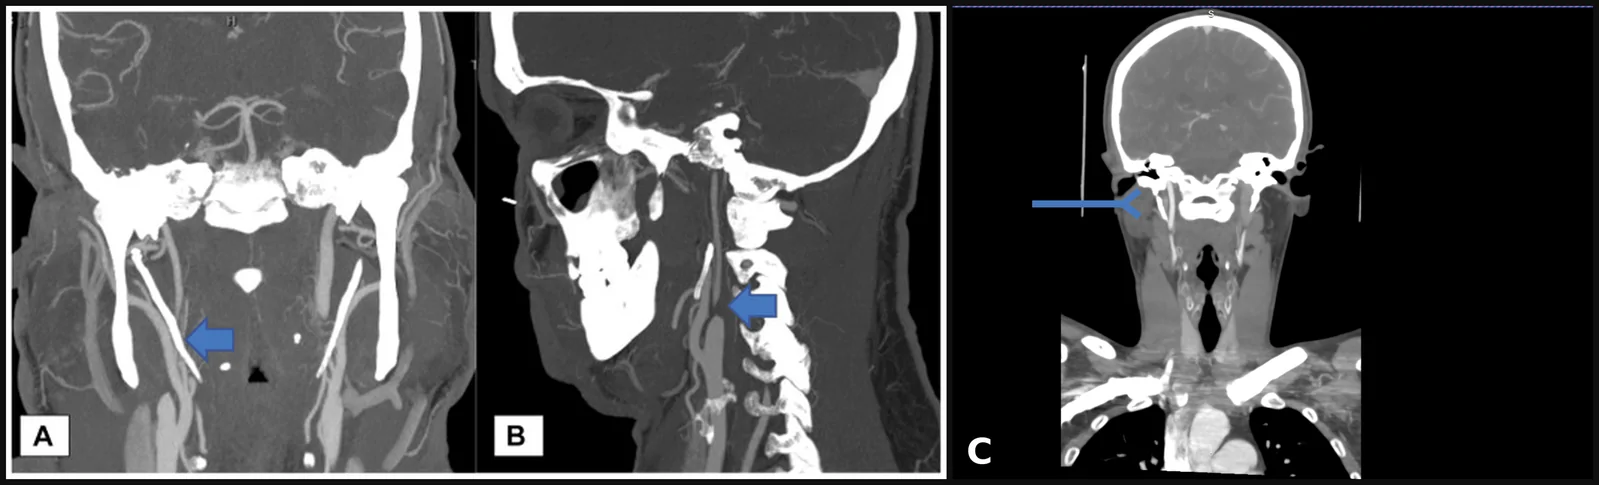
CT angiography of the head and neck demonstrating left internal carotid artery (ICA) dissection in the context of vascular Eagle syndrome (stylocarotid syndrome) CT angiography of the head and neck demonstrating left ICA dissection. (A) Coronal maximum intensity projection (MIP) view showing occlusion of the left ICA from the level of the common carotid bifurcation with progressive luminal tapering (arrow). Note the asymmetry between the patent right ICA and the occluded left side. (B) Sagittal reconstructed view confirming left ICA dissection with luminal irregularity and near-complete occlusion at the carotid foramen (arrow). Re-opacification of the distal left ICA territory was demonstrated via the left posterior communicating artery. (C) Coronal view at the level of the skull base demonstrating the elongated left styloid process in close proximity to the affected ICA segment (arrow), consistent with vascular Eagle syndrome (stylocarotid syndrome) as a contributing mechanical factor.
